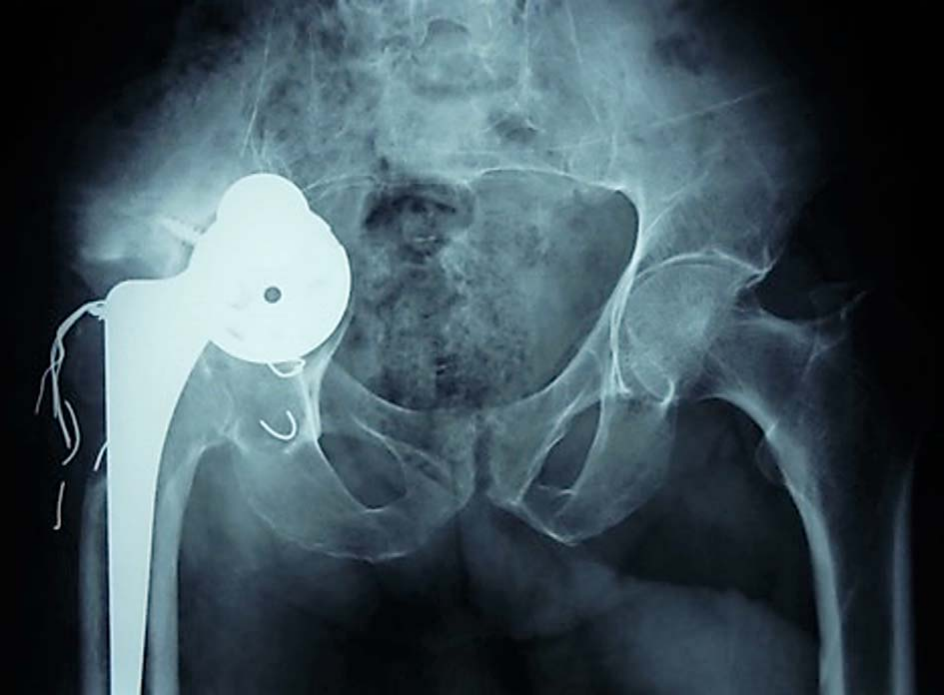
Anteroposterior X-ray of the pelvis showing acetabular component migration medial to Kohler’s line with proximal migration of the stem.
